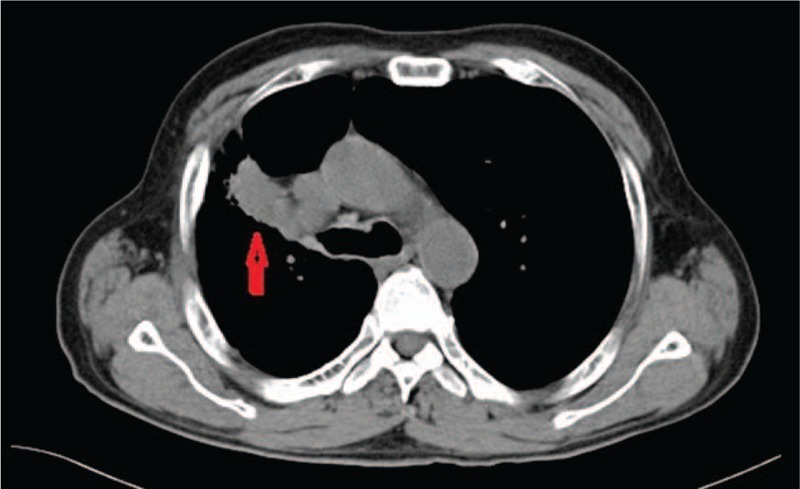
Chest computed tomography (CT) indicates a lesion in the right lung.
